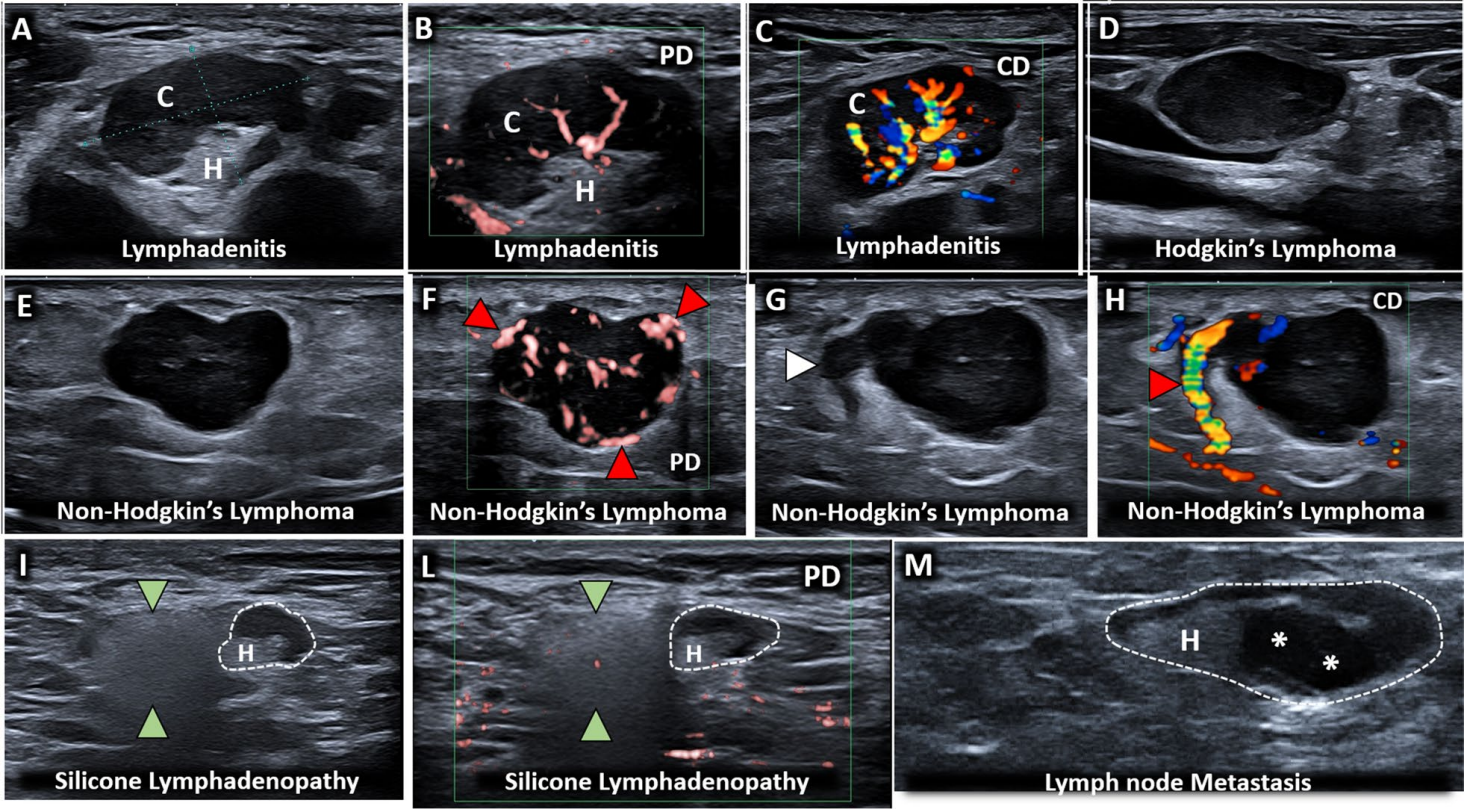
A glance-in-depth at the multiparametric sonographic assessment of lymph nodes. Benign lymphadenopathy (A) usually preserves the hyperechoic hilum *(H)*, the hypoechoic cortex *(C)* and a unipolar vascularization with feeding vessels branching from the hilum to the cortex (B, C) Instead, a disappearance of the hilum (D), a rounded/globular shape (E) and a multipolar vascularization (F) with feeding vessels *(red arrowheads)* originating from the peripheral portion, can be considered the most common sonographic findings of malignant lymphadenopathy. Of note, considering the extreme variability of sonographic patterns of lymph nodes, several “atypical” findings can be identified in daily practice—e.g., serpiginous protrusions *(white arrowhead)* related to ectatic vessels *(red arrowhead)* (G, H) a normal lymph node *(white dotted line)* coupled with a “snowfall” lymph node *(green arrowheads)* due to particle depositions (I, L) eccentric thickening of the cortex *(white asterisks)* with lateral displacement of the hilum *(H)* (M). PD: power doppler; CD: color doppler
